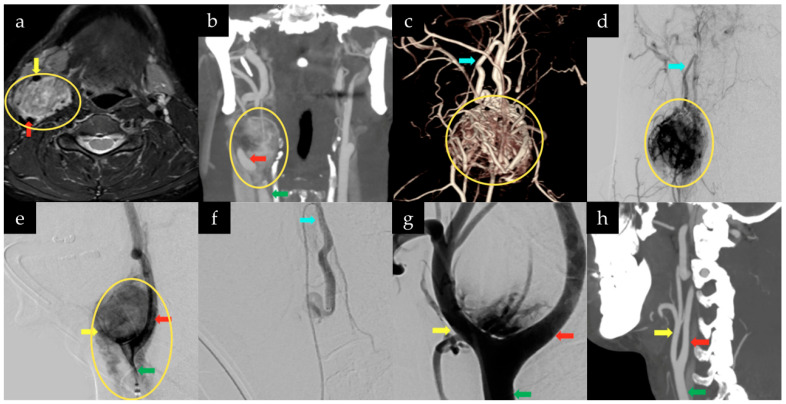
Multimodal imaging of a 42-year-old man with a Shamblin Type 3 right CBT treated with preoperative embolization and microsurgical resection. (a) Axial contrast-enhanced MRI demonstrates a hypervascular mass (yellow circle) at the right carotid bifurcation with displacement of the internal carotid artery (ICA, red arrow) and external carotid artery (ECA, yellow arrow). (b) Coronal CT angiography shows separation of the ICA (red arrow) and ECA (yellow arrow) by the tumor (yellow circle), with the common carotid artery (CCA, green arrow) identified proximally, consistent with circumferential carotid encasement. (c–e) Three-dimensional CT angiographic reconstruction and digital subtraction angiography demonstrate extensive tumor (yellow circle) related neovascularity arising predominantly from external carotid artery branches, including the ascending pharyngeal artery (blue arrow), with circumferential involvement of the ICA, ECA, and proximal CCA. (f,g) Selective and common carotid artery angiography following embolization demonstrate marked reduction in tumor vascularity with preserved patency of the ICA, ECA, and CCA. (h) Three-month postoperative CT angiography confirms complete tumor resection with preserved carotid artery anatomy and no evidence of residual or recurrent disease.
